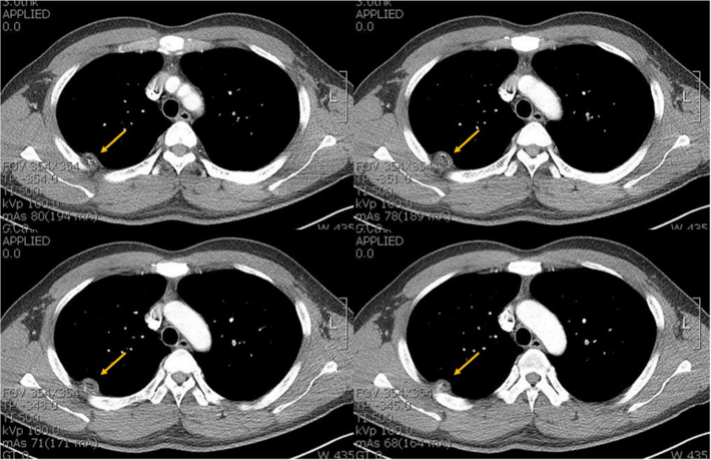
Contrast-enhanced computed tomography (CT) demonstrates the intrathoracic fatty mass with calcification that has obtuse margin projecting into the right hemithorax (arrow).
